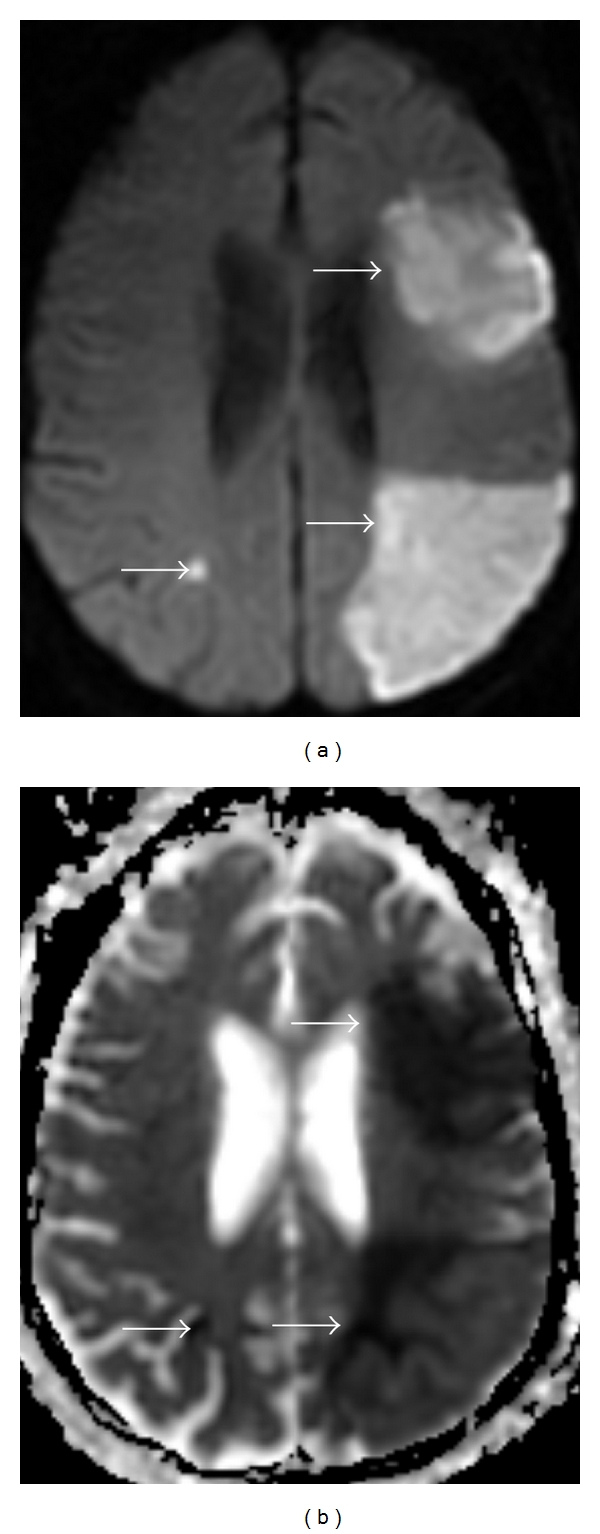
A 76-year-old female with history of acute myelogenous leukemia and atrial fibrillation, presenting with altered mental status related to embolic stroke. (a) DWI sequence. (b) ADC map. Areas of signal hyperintensity on the DWI sequence (arrows) are confirmed to represent restricted diffusion by the signal hypointensity on the ADC map (arrows).
